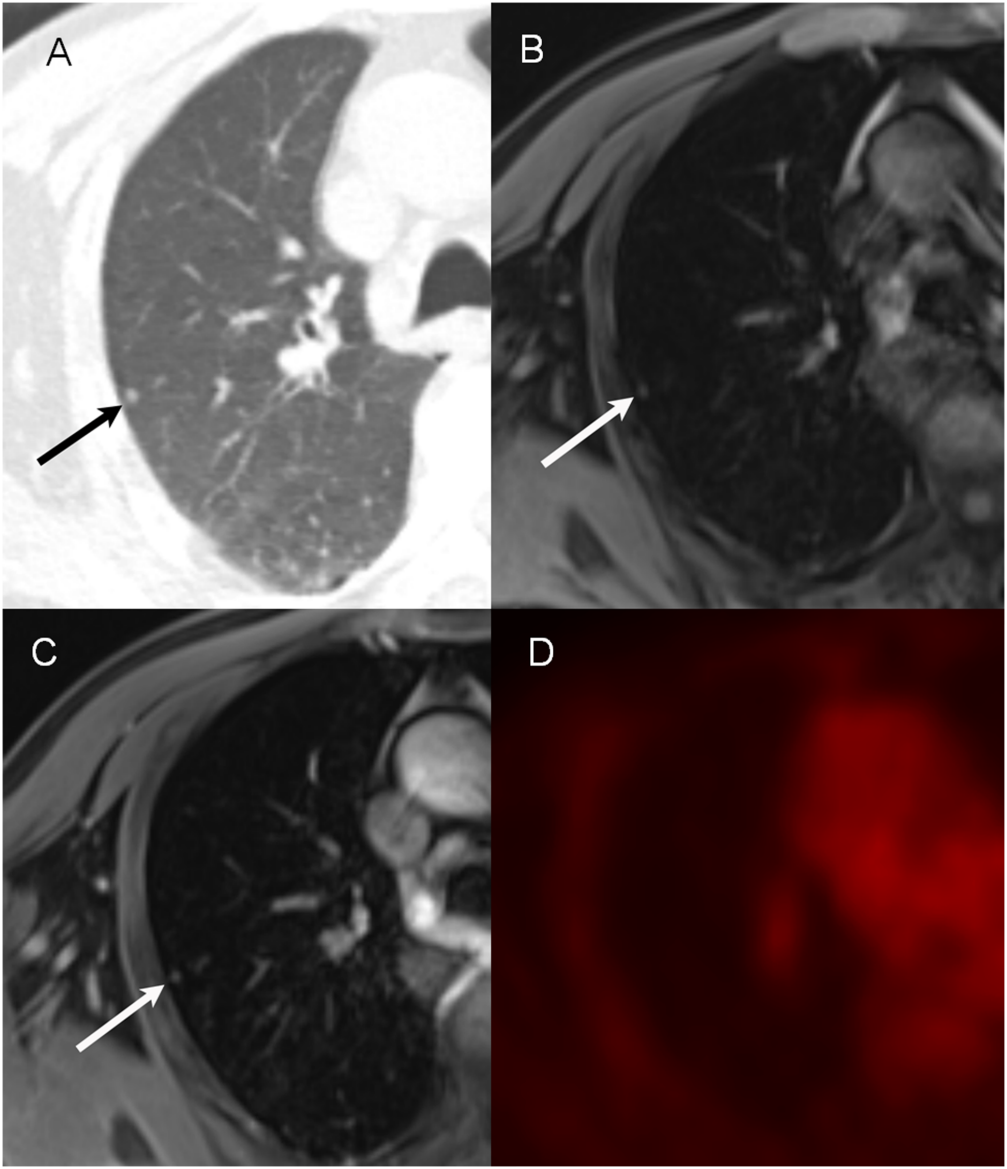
An example of the non-FDG-avid nodule on PET/MR images (68-year-old man with lung cancer). On CT image (A), a tiny nodule (3 mm) is identified (arrow) in the right upper lobe. This lesion is also visible on pre-contrast VIBE (B) and post-contrast VIBE (C) images. Both readers identified this nodule during both the first and second sessions. However, this lesion is not visible on PET (D) images due to non-FDG-avidity.
